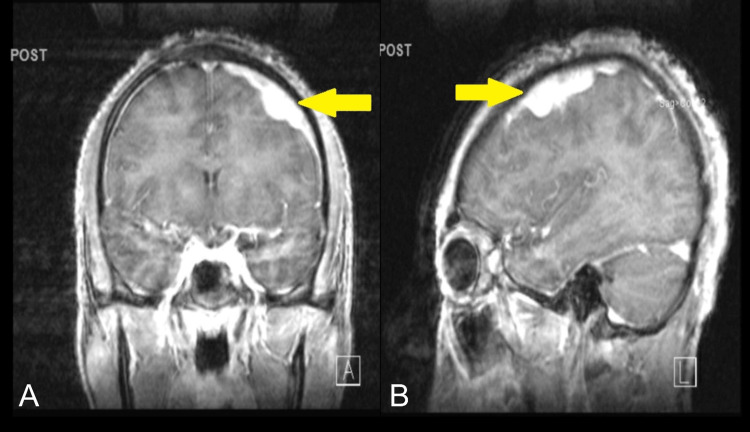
Brain MRI image of extra-axial meningeal mass. The images show (A) extensive abnormal extra-axial soft tissue density measuring 14 mm in maximum depth over the left cerebral convexity in post-contrast coronal T1-weighted brain MRI image and (B) sagittal view of the T1-weighted brain MRI.
